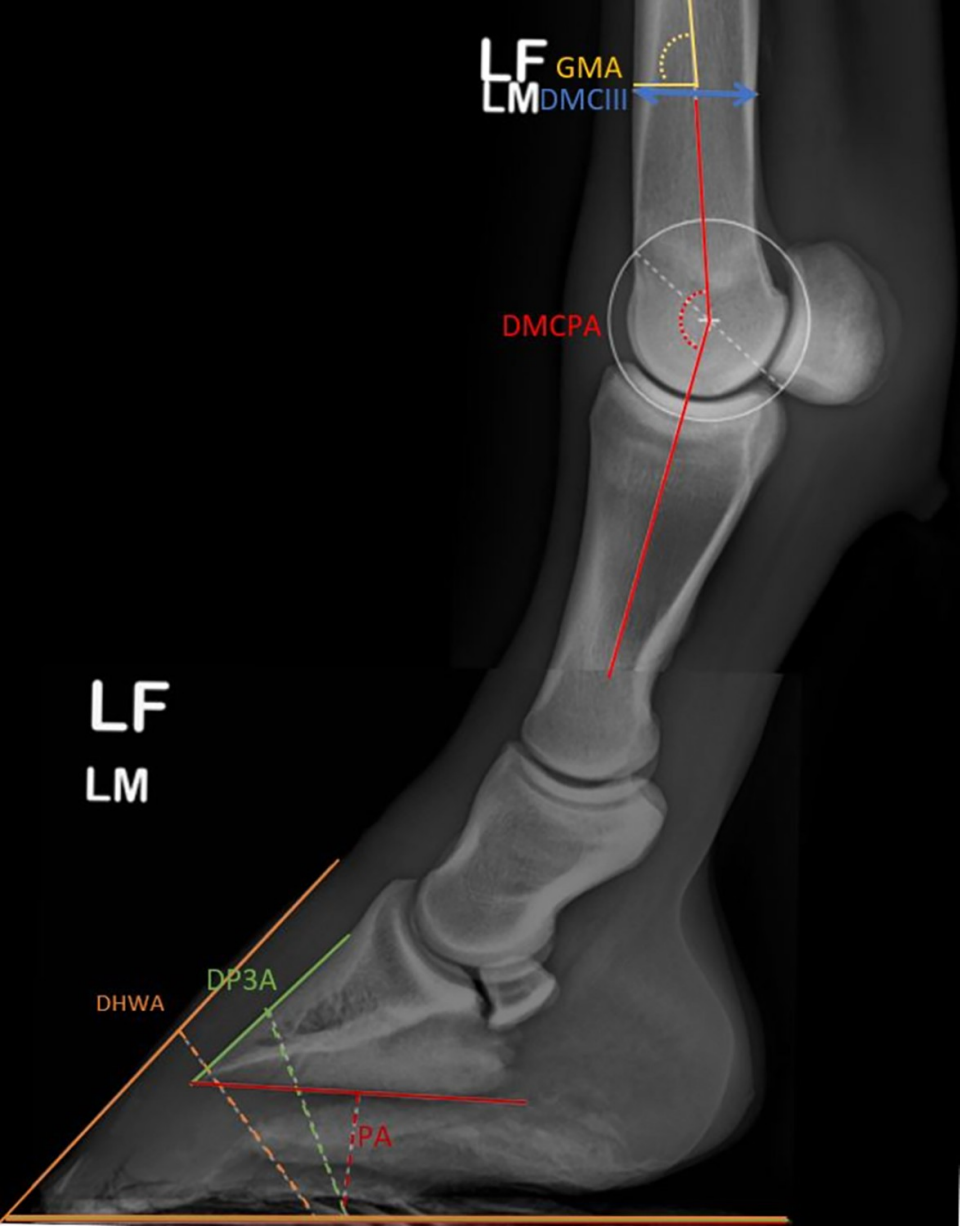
Latero-medial radiographs of an individual’s left foot and fetlock, showing the different measurements performed. GMA: Ground to third Metacarpal bone Angle; DMCIII: Diameter of the third Metacarpal bone; DMCPA: Dorsal Metacarpophalangeal Angle; DHWA: Dorsal Hoof Wall Angle; DP3A: Dorsal Third Phalanx Angle; PA: Palmar Angle.
